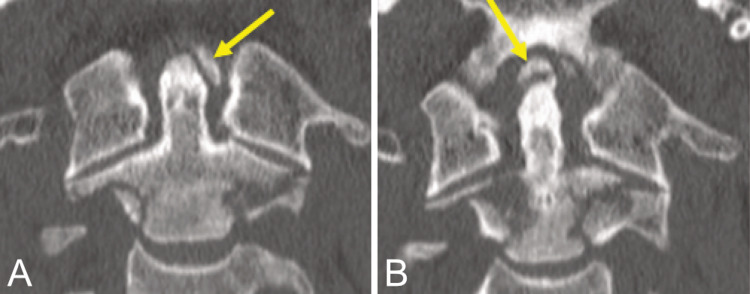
Computed tomography scans (A) Left alar ligament avulsion fracture (yellow arrow) and (B) apical ligament avulsion fracture (yellow arrow).
